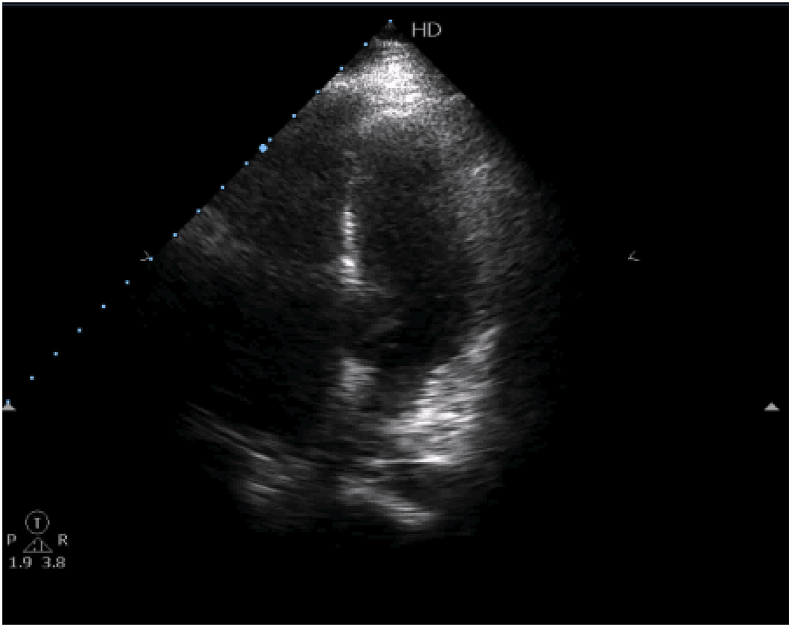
2D Echo Cardiography showing apical four chamber view (Post Operative).
